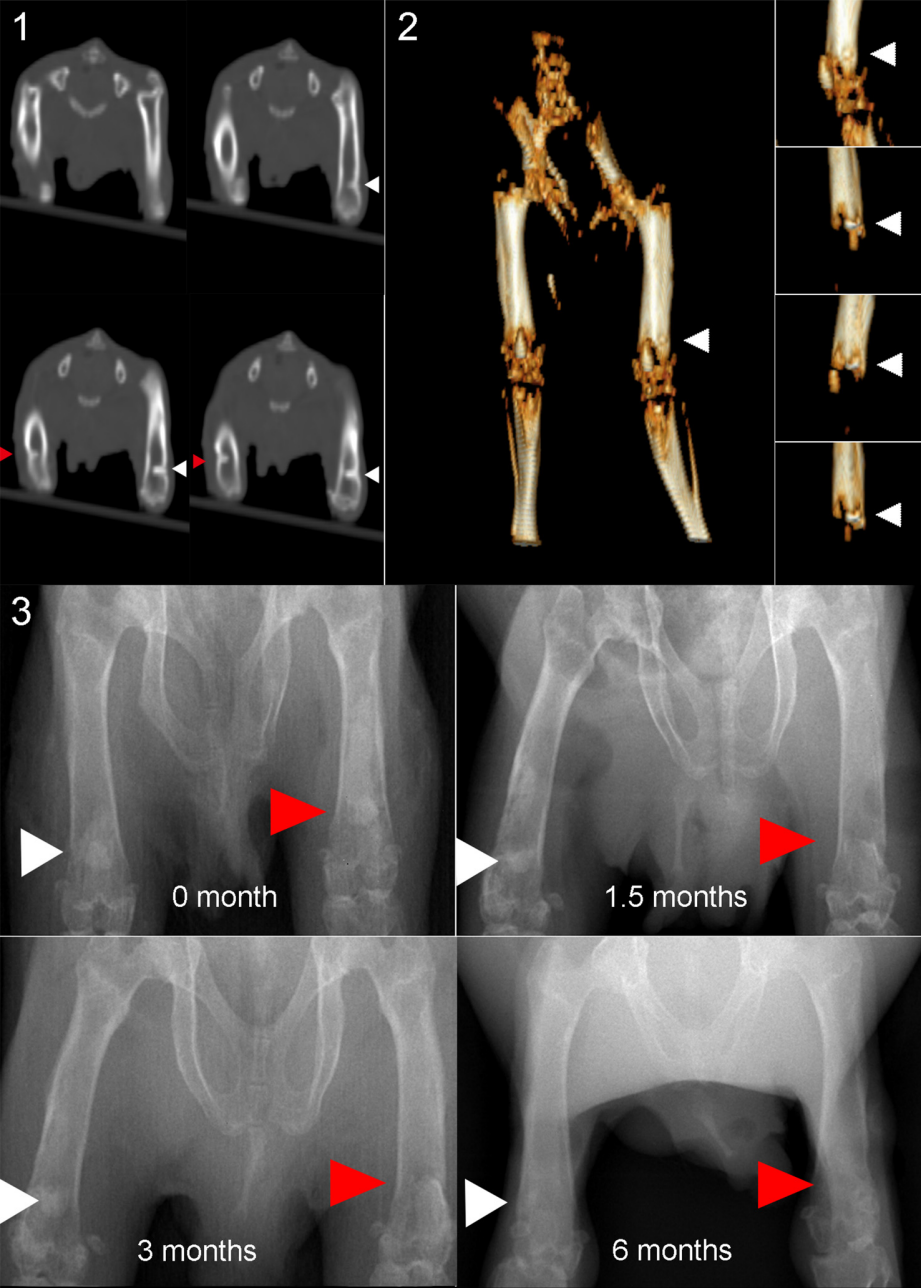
Computed tomography (CT) and radiography images showing cement sample integration with the host bone; a) CT image taken immediately after operation; b) 3D reconstructed CT image immediately after operation; c–f) radiographs taken 0, 1.5, 3, and 6 months, respectively.
